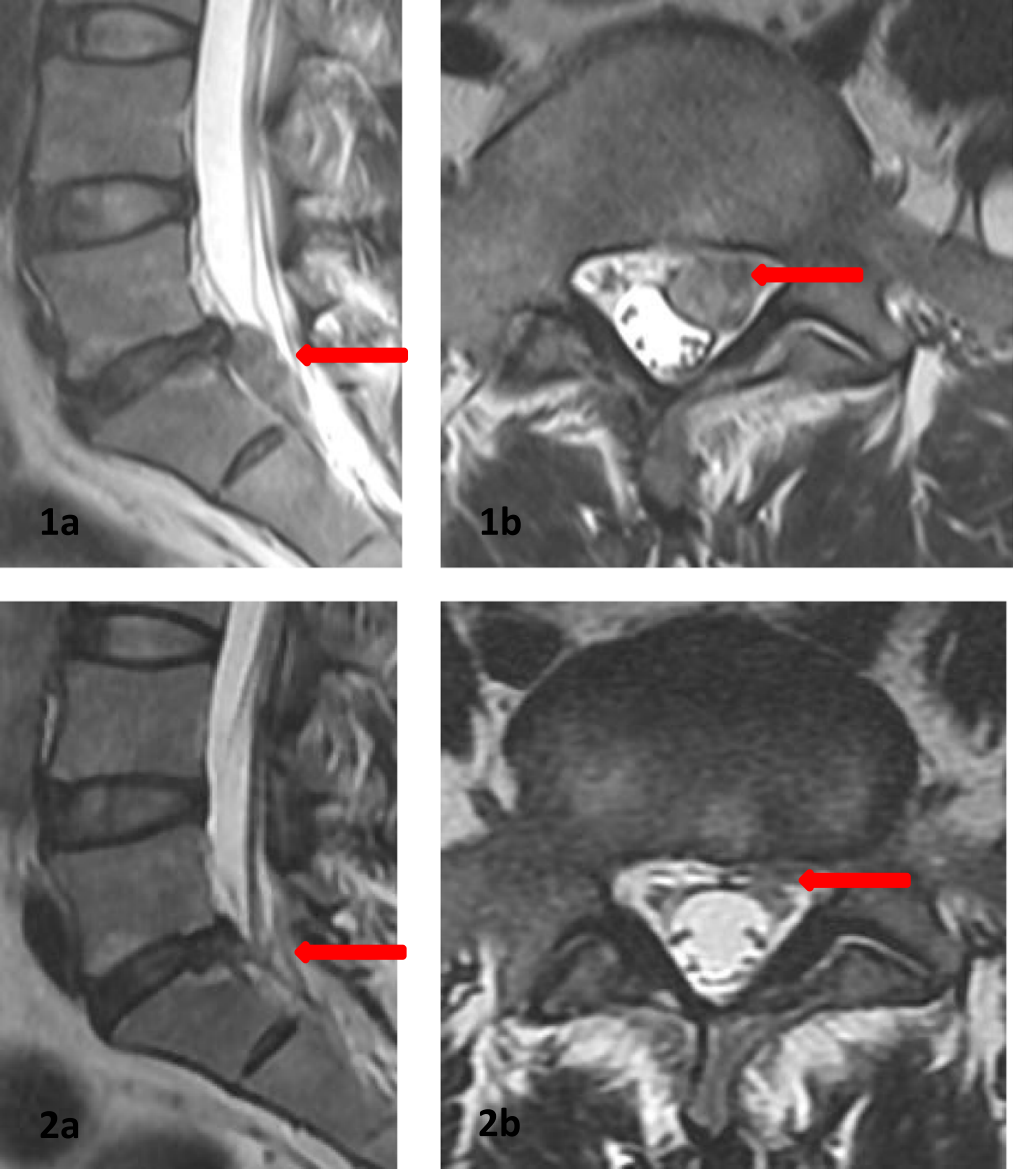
Male patient, 41 years old, with low back pain with referred left leg pain for 3 weeks. MRI indicates that a huge ruptured lumbar disc herniation on the left at level L5/S1. 1a and b: The baseline volume of the protrusion is 3307.0 mm^3^. SLRT: left 15^o^, right 60^o^, JOA score: 49. 2a and b: The volume of the protrusion was 486.46 mm^3^ at the last follow-up. SLRT: left 70^o^, right 70^o^, JOA score: 5
